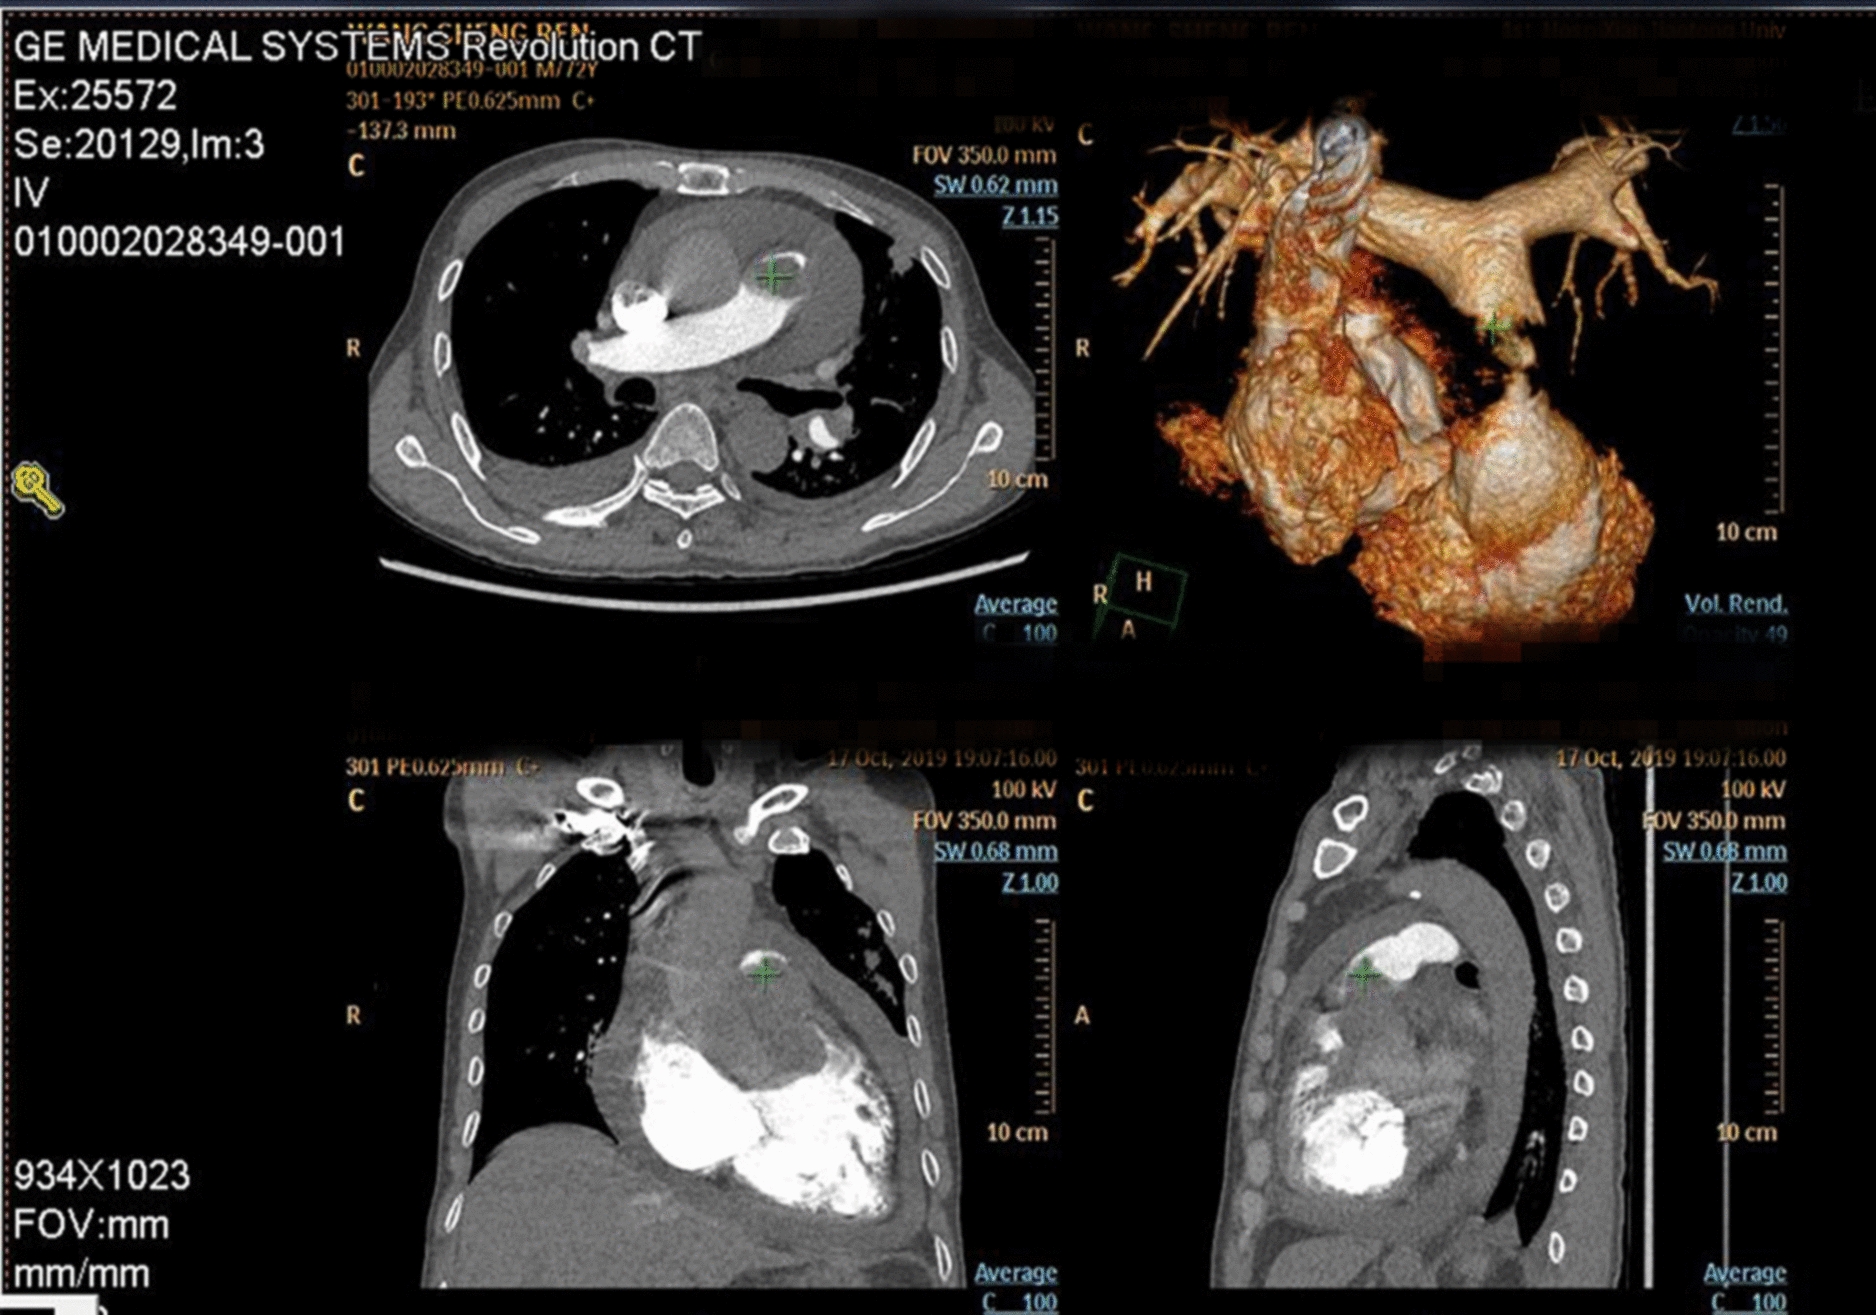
Computed tomography angiography (CTA) findings of superior vena cava images acquired in multiple planes. Arrow indicates the location of the lesion
